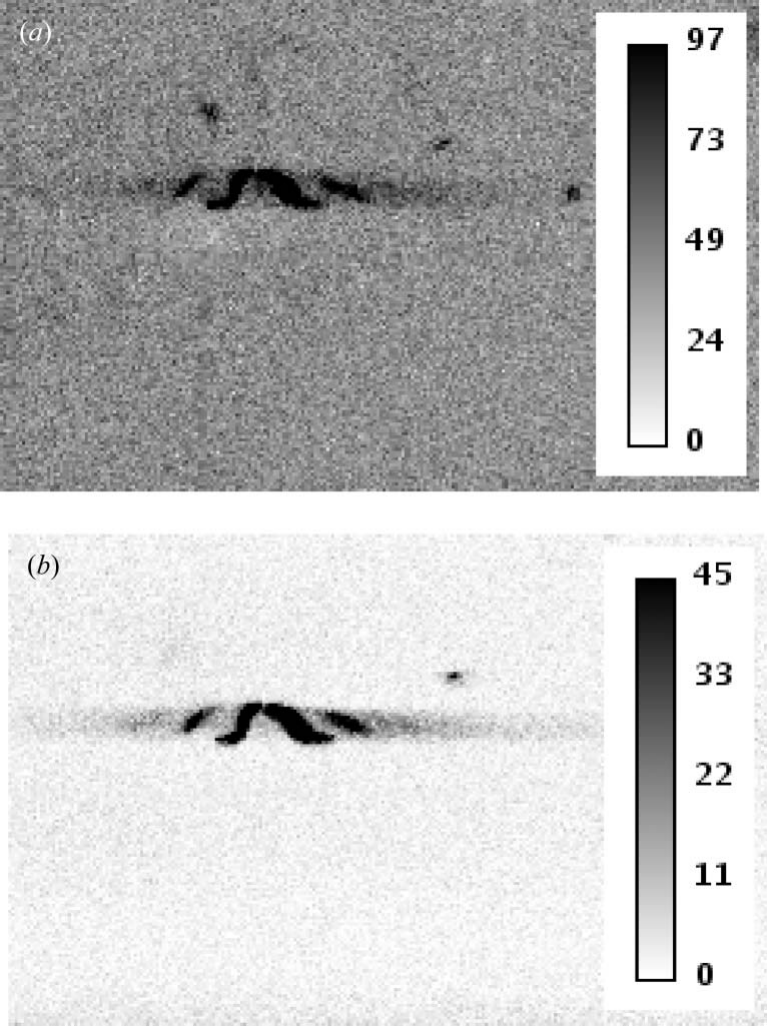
Detector image (zoom) of the 201 signal of NdGaO_3_ using 15.92 keV X-rays and a global threshold of (*a*) 9.5 keV and (*b*) 12 keV.
